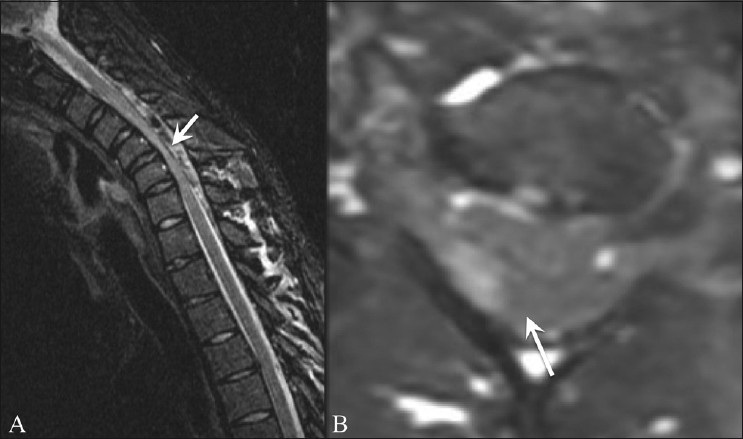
Sagittal flexion (A) and axial (B) 3D fast imaging employing steady-state acquisition magnetic resonance images show prominent flow voids within the dorsal epidural space (arrows) with asymmetric cord compression
